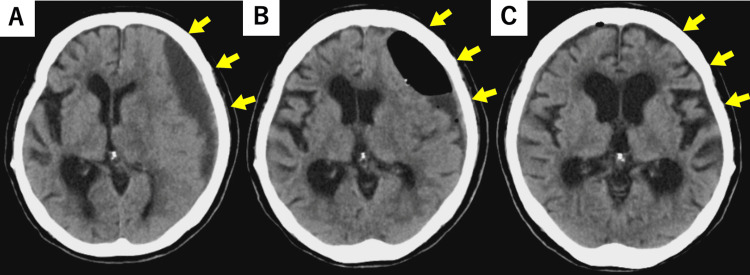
A: CT on the eighth day of admission (third day after the addition of the new valve); B: 10th day of admission (fifth day after the addition of the new valve); C: CT on the 25th day of admission (15th day after hematoma cleaning) A: The ventricle had enlarged over time, but the hematoma remained. B: CT immediately after hematoma cleaning C: The hematoma had almost disappeared.
